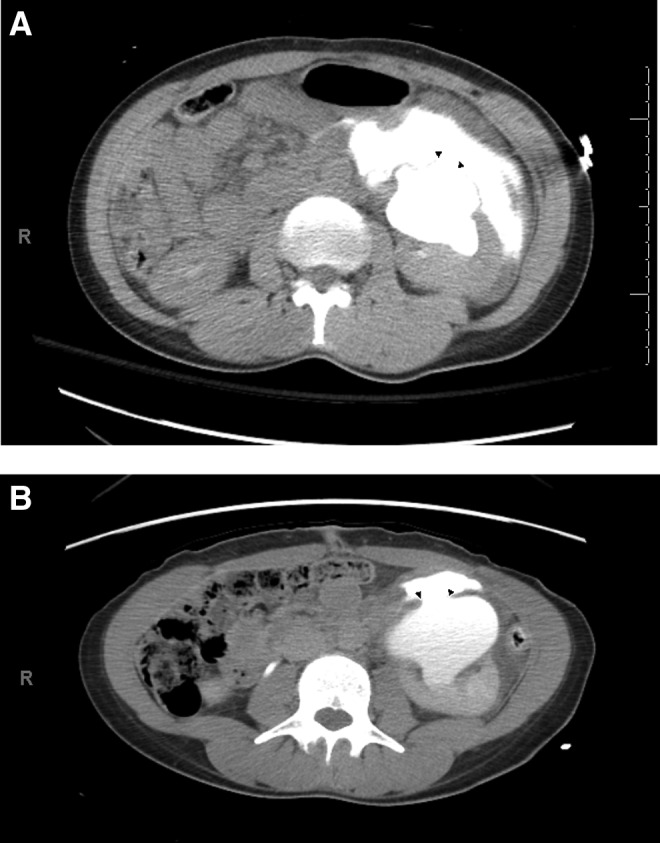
(A) Delayed phase of CT urogram; axial image of left kidney showing contrast extravasation and 11 mm defect (*arrow heads*) in the renal pelvis. (B) Delayed phase of CT urogram; axial image of left kidney showing persistence of pelvic defect (*arrow heads*) with contrast extravasation, but marked interval decrease in urinoma after percutaneous nephrostomy tube placement.
